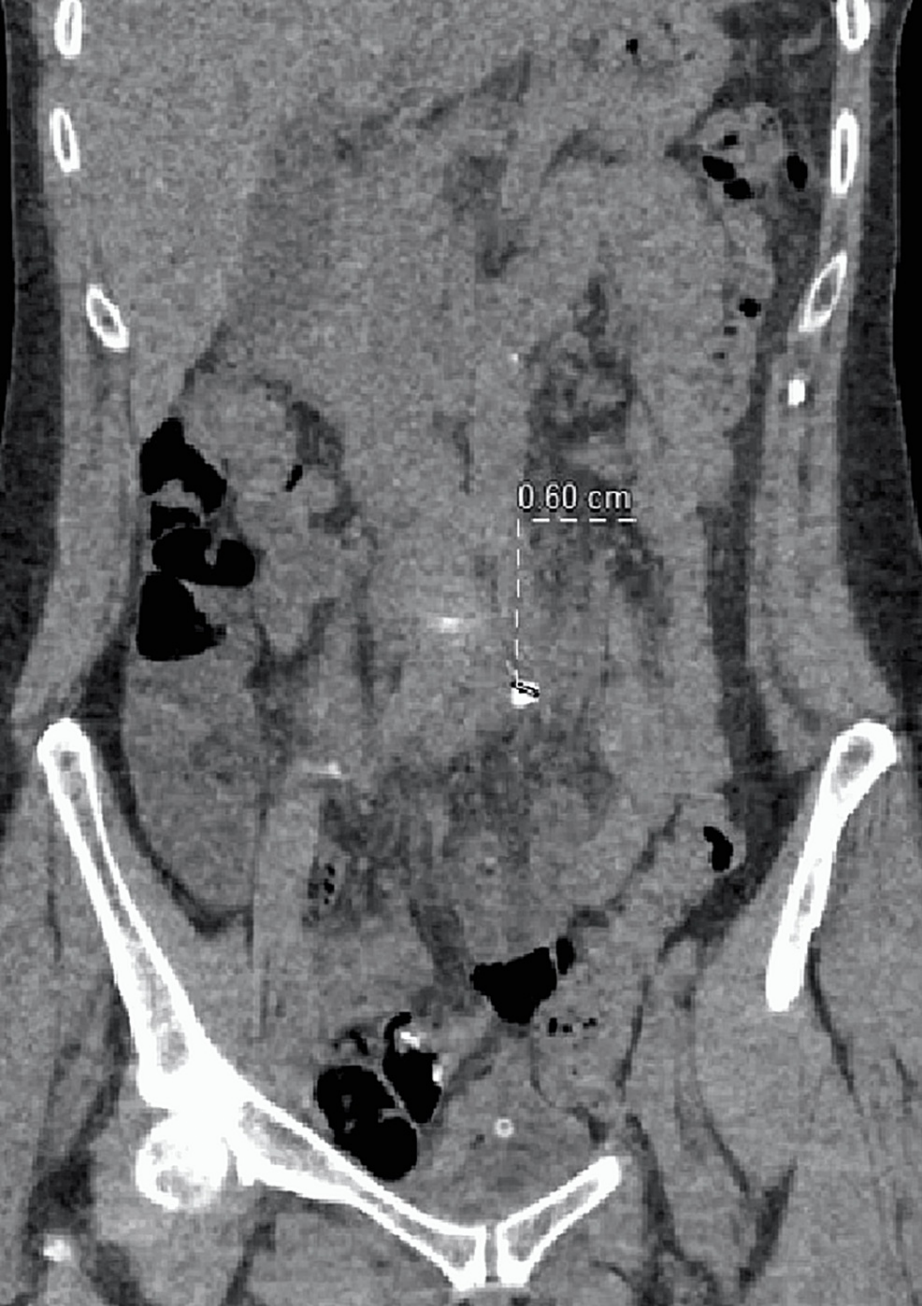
CT-abdomen, native sequence, ureteral stone in the medial portion of the left ureter (6-mm, 1100 Hounsfield units), coronal view.
